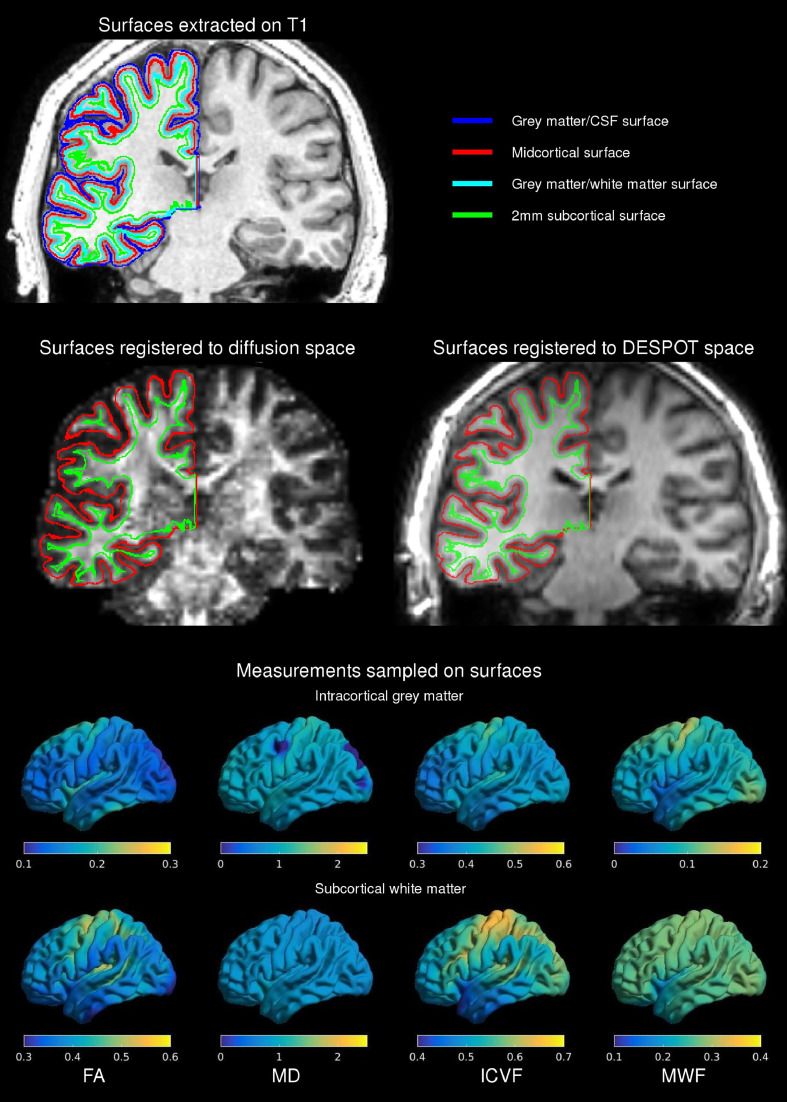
Image processing framework. Grey matter-white matter and grey matter-CSF cortical surfaces were extracted from the T1-weighted image (top, cyan and blue respectively) and midcortical and 2 mm subcortical surfaces were generated using a Laplacian potential (top, red and green respectively). These surfaces were registered to diffusion and DESPOT space using the FA image (middle left) and IR-SPGR (middle right) respectively. Measurements from diffusion and DESPOT scans were sampled along these surfaces (bottom). Examples of mean values in patients on the two surfaces are shown for diffusion (FA, MD, ICVF) and DESPOT (MWF) scans.
